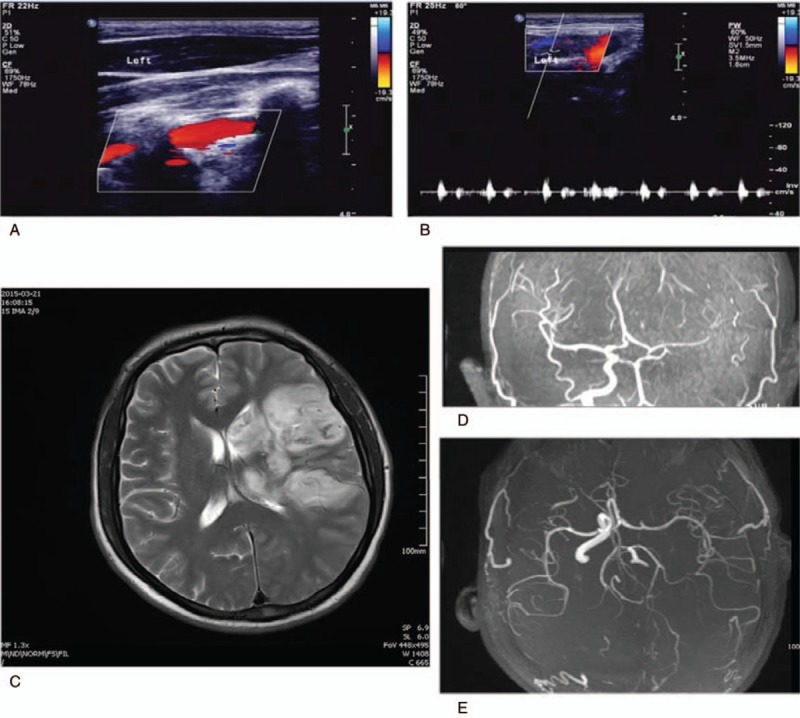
Carotid ultrasonography and cranial magnetic resonance imaging (MRI). (A) Left carotid artery was occluded and lacked blood flow; (B) Blood flow signals not detected in the left carotid artery; (C) Cranial MRI showing a new large cerebral infarction in the left hemisphere; (D, E) The left internal carotid artery is occluded, right internal carotid artery supplies compensatory blood to the left anterior cerebral artery and middle cerebral artery through the anterior communicating artery, and distal branches of the left middle cerebral artery are poorly visualized.
